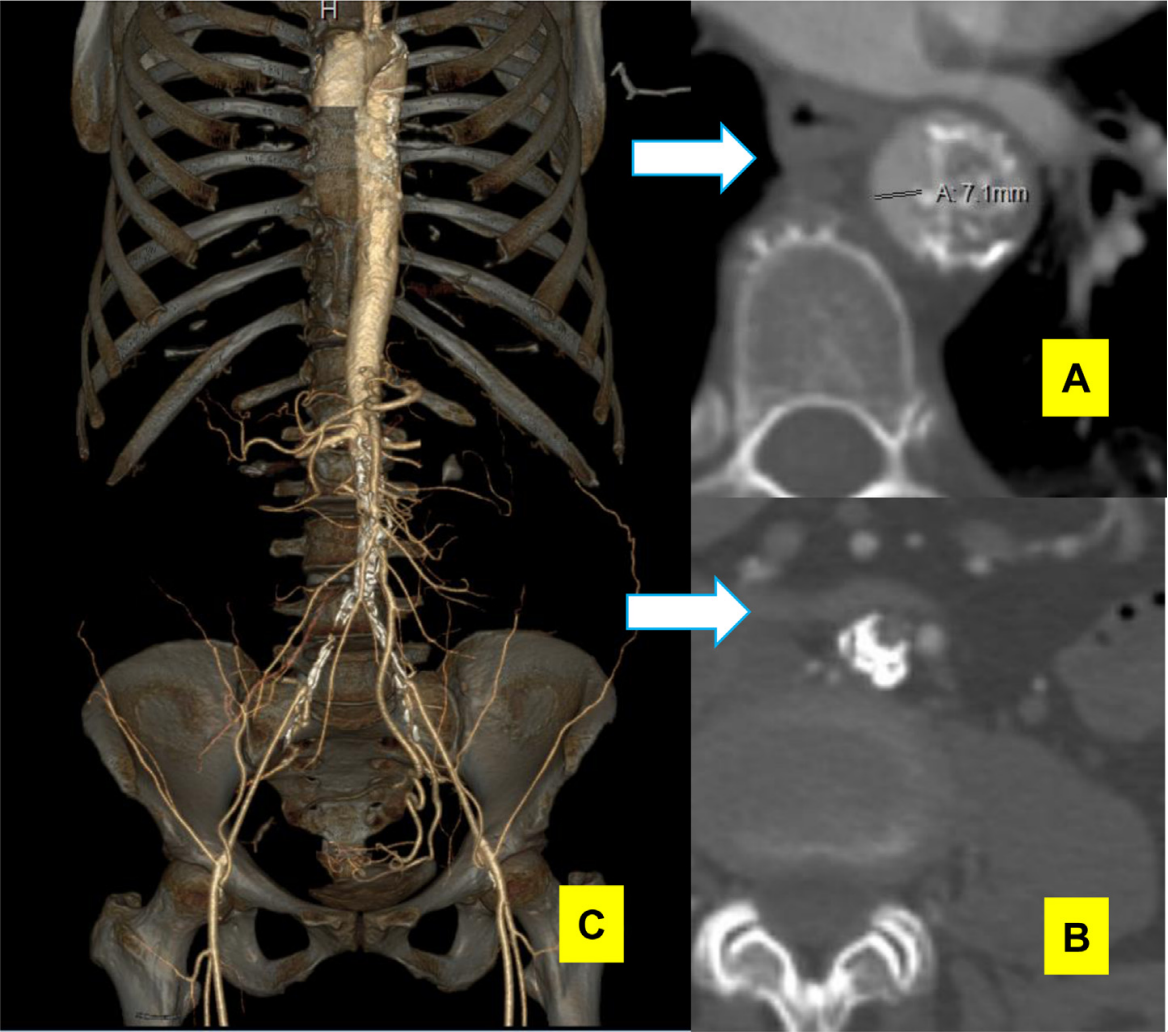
Computed tomography angiogram (CTA) showing severely stenotic aortic segment (A), total occlusion of the distal abdominal aorta (B), and mid-visceral aortic atherosclerosis (C).
